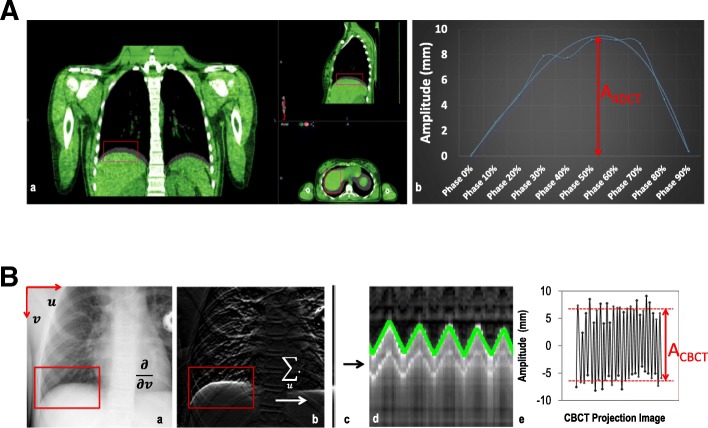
a Left) Rigid registration of the cranial-caudal position of the right diaphragm (inside the red box) in all breathing phases. Right) The difference between the most extreme translations was defined as the amplitude (A_4DCT_). b Amsterdam Shroud (AS) method to manually track the CC diaphragm position in CBCT projection images. a) Region of interest (red box). b) CC gradient filter applied and sum of all pixels creates a 1D image. C) This is repeated for all projection images, creating a 2D image. d) Detection of diaphragm positions in inhale and exhale breathing phases. e) Pixel coordinates translated to CC position. The amplitude was defined as the displacement between averaged end-inspiration and end-expiration diaphragm positions (A_CBCT_)
